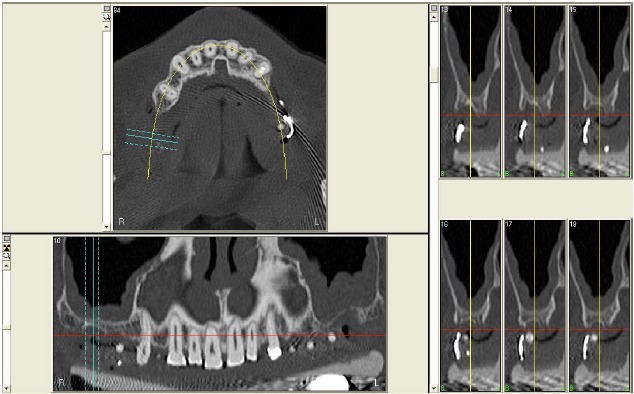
CT scan of the upper maxilla with severe posterior bone atrophy.
